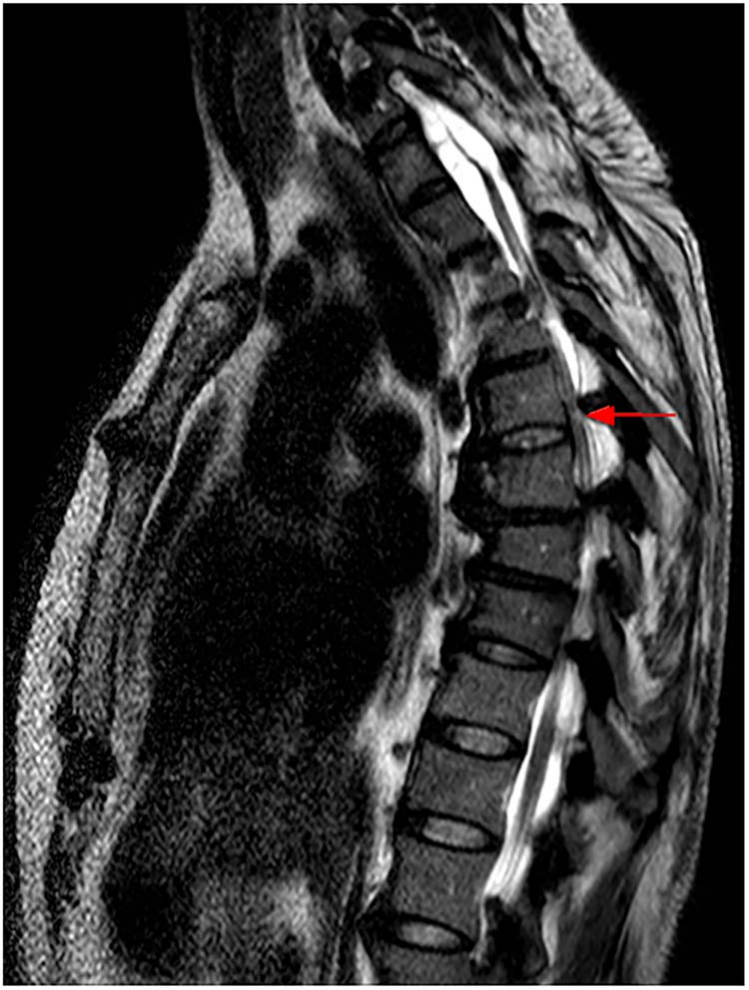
MRI scan of the thoracic spine at the time of admission, with hyperintense intramedullary T2 signal at Th6, indicating myelopathy (red arrow).
